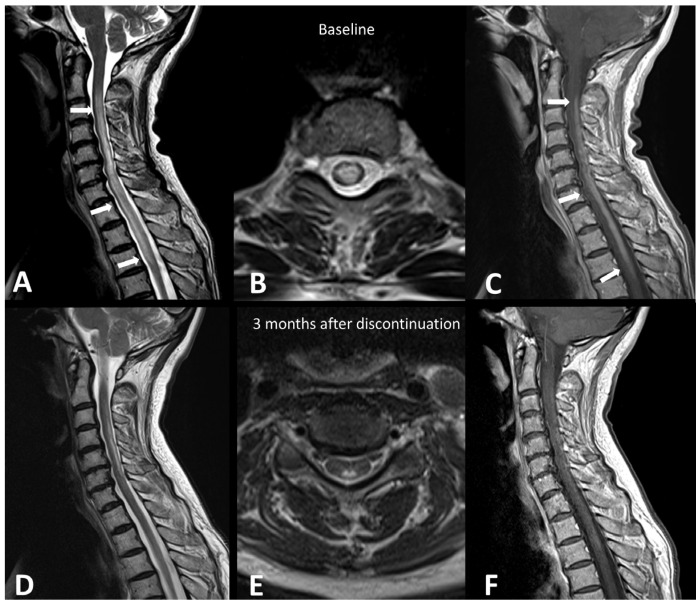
Immuno-related extensive myelitis in a patient suffering from left arm melanoma. The patient had diffuse sensitive symptoms in the forearms a few weeks after the beginning of antiPD-1 treatment. Medullary MRI revealed a T2 hypersignal on the T2 sequence involving more than three vertebrae on the sagittal plane (arrows in (A)) and more than 50% of the medullary diameter on the transverse plane (B). An extensive medullary enhancement is also seen (arrows in (C)). The treatment was suspended, and the patient was treated with intravenous immunoglobulin and prednisone. Medullary MRI performed 3 months after ICI discontinuation showed partial regression of T2 hyperintensities (D,E) as well as almost complete regression of the medullary enhancement (F).
